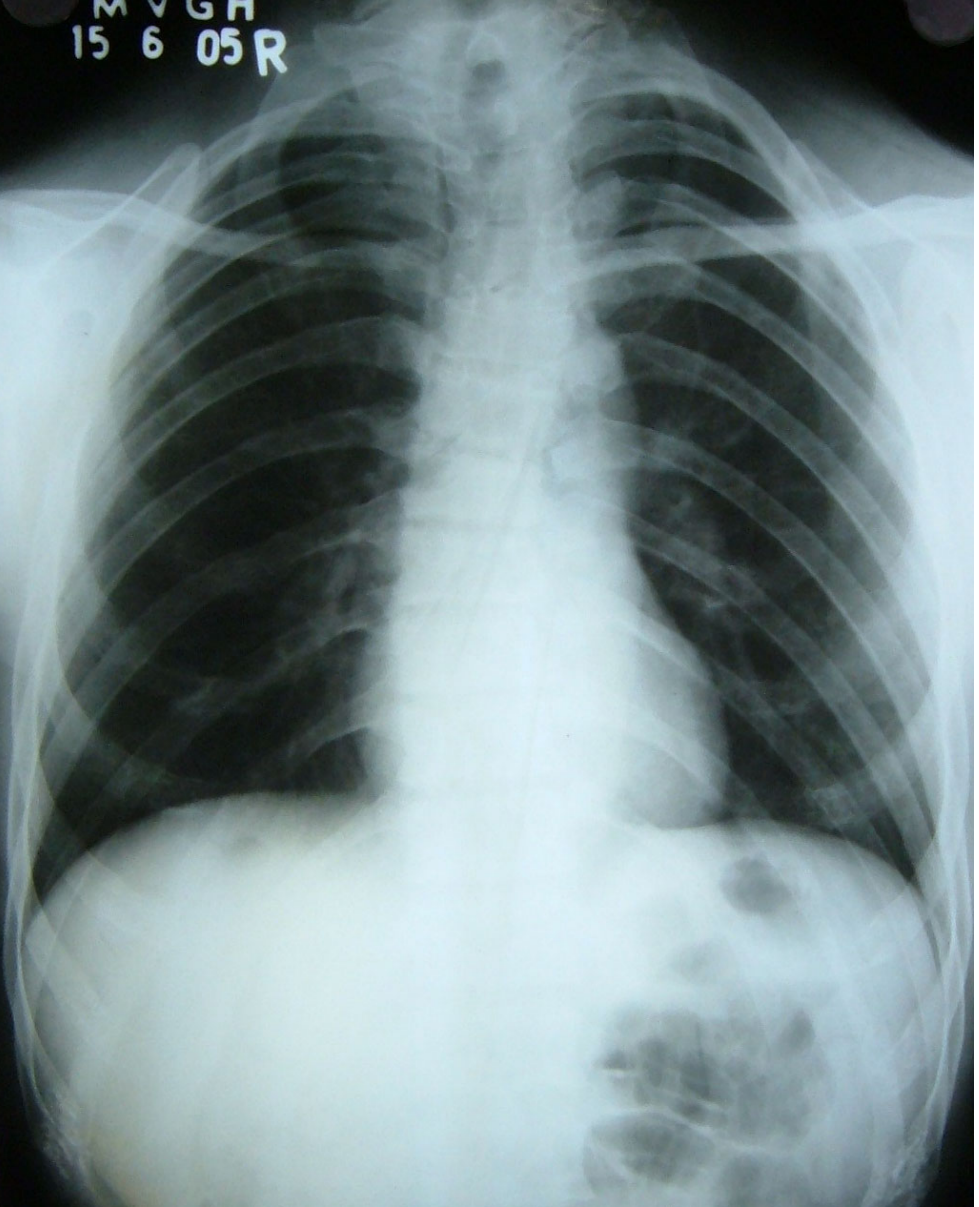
Chest radiograph (PA View) of the patient showing thinning and hypoplasia of the clavicles and bell shaped rib-cage.
